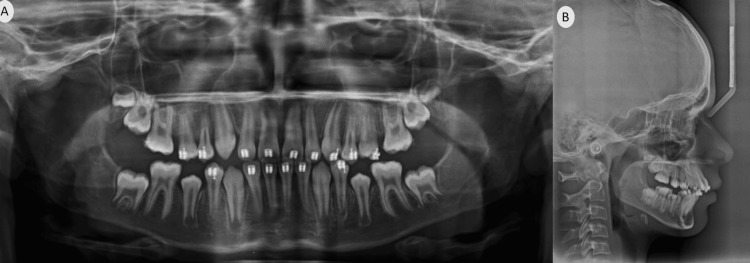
Post-Quad Block radiographs (A) Orthopantomogram; (B) Lateral cephalogram
